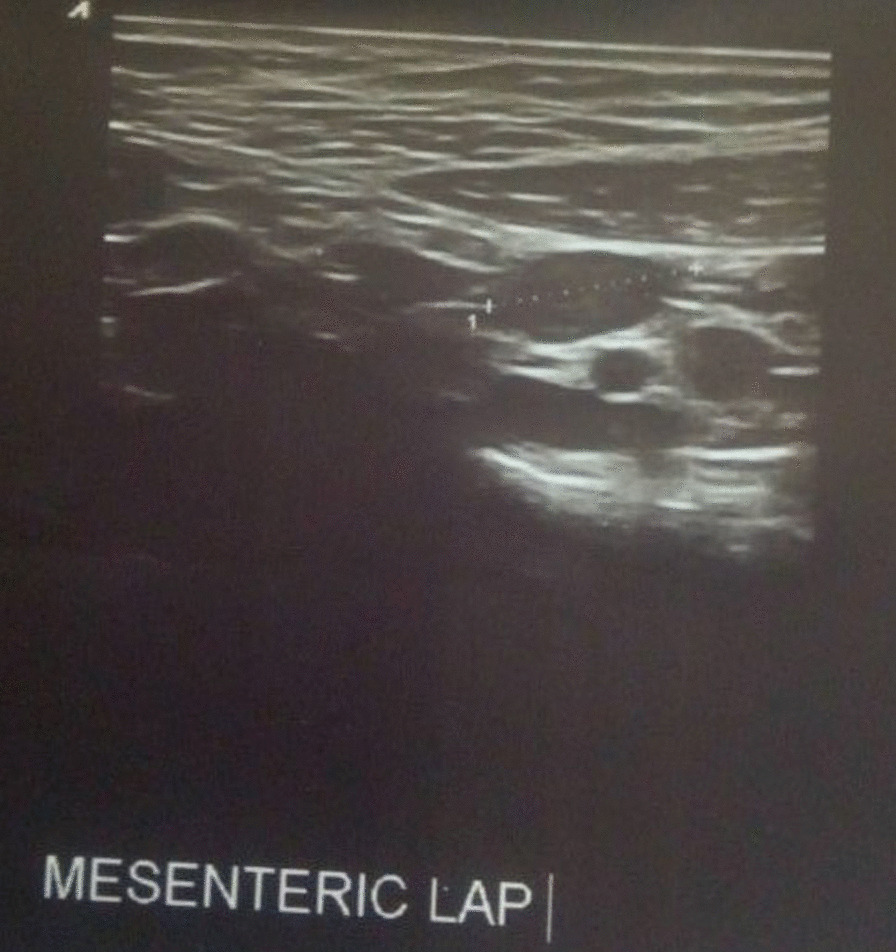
Abdominal ultrasound image showing multiple mesenteric lymphadenopathies
